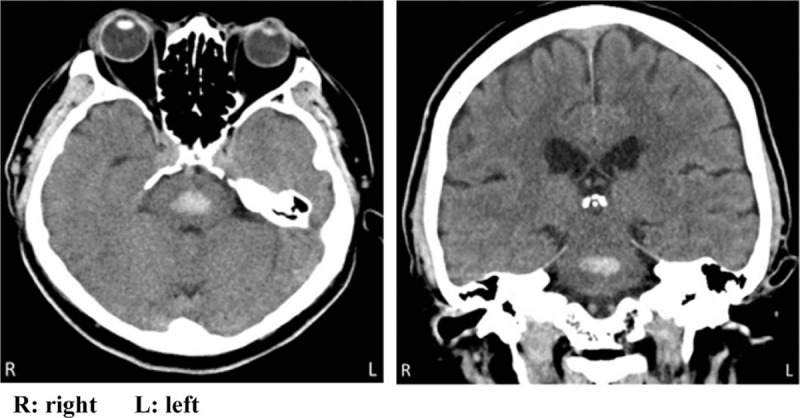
The axial and coronal images of the initial brain computed tomography showed an acute phase hemorrhage (volume 1.5 mL) of the central pons.
